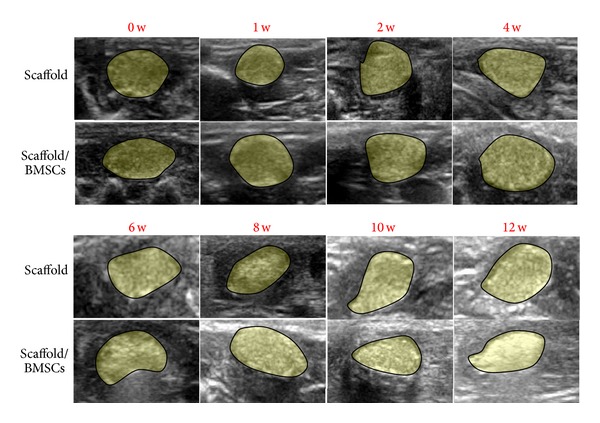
Ultrasound images of implanted scaffold over time, showing the evolution of constructs of the two groups (scaffold or scaffold/rBMSCs). The ROIs were signed by translucent yellow overlays.
